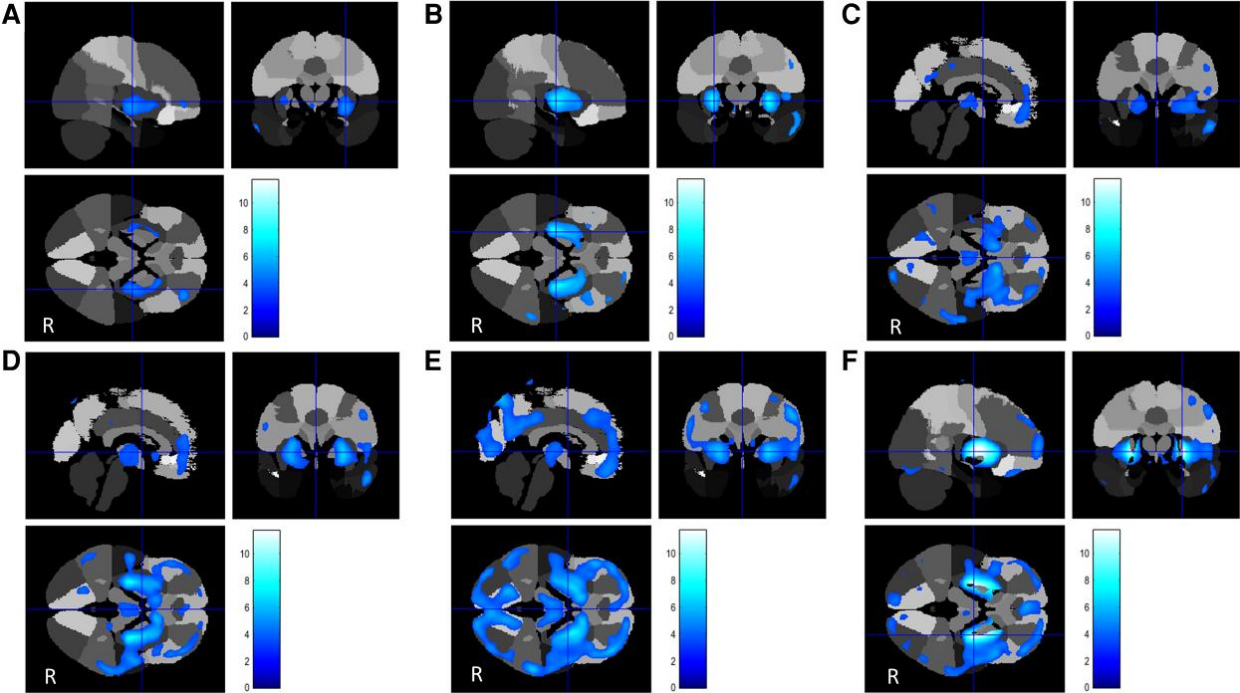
FDG uptake compared to controls in inherited dystonias that only showed hypometabolism (excluding PANK2). Areas of relative regional hypometabolism are displayed in a blue-scale with brighter tones indicating higher t-scores. (A) TOR1A (*n* = 7): Putamen-frontal hypometabolism. (B) THAP1 (*n* = 3): Caudate-putaminal and right fronto-parietal hypometabolism. (C) SGCE (*n* = 6): Head of caudate, globus pallidus, inferior frontal lobe hypometabolism. (D) KMT2B (*n* = 7): Marked caudate-putaminal (striatal) and bi-frontal and bi-parietal hypometabolism. (E) HPRT1 (*n* = 5). Extreme caudate-putaminal-pallidal, medial thalamic, and pan-hemispheric and antero-superior cerebellar hypometabolism. (F) GCDH (*n* = 9). Relative regional hypometabolism in the posterior putamina and globi pallidi. All results are displayed on the 97-region version of the maximum probability atlas derived from the Hammers Atlas Database^33–35^ (www.brain-development.org/brain-atlases), which is a 3D maximum probability atlas of the human brain.
